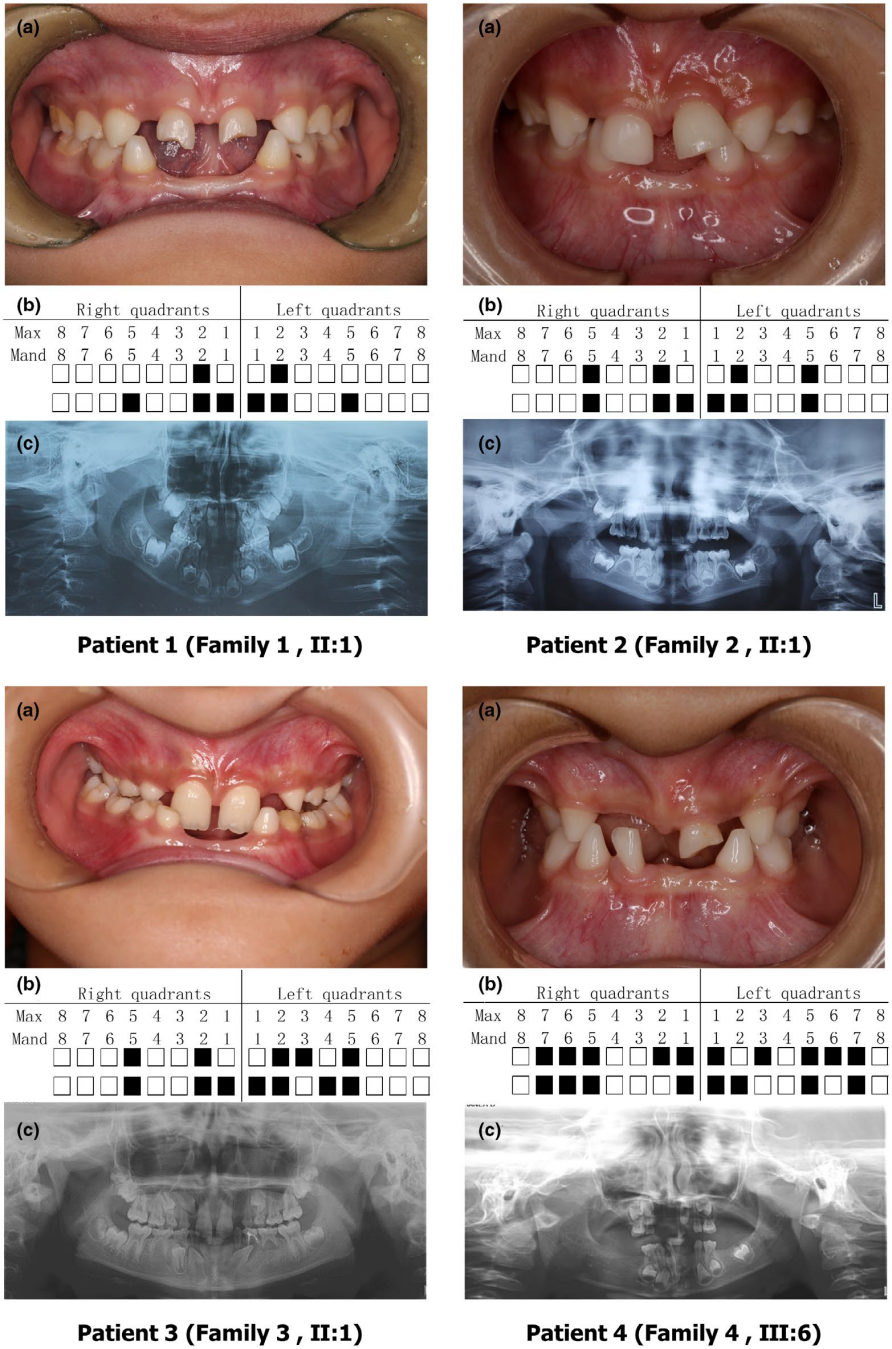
Characteristics of the four probands with non‐syndromic tooth agenesis (NSTA). (a) Intraoral image of probands; (b) Legend of intraoral missing teeth of probands; (c) Panoramic radiography of probands. Black squares indicate missing teeth; Max: maxillary; Mand: mandibular
